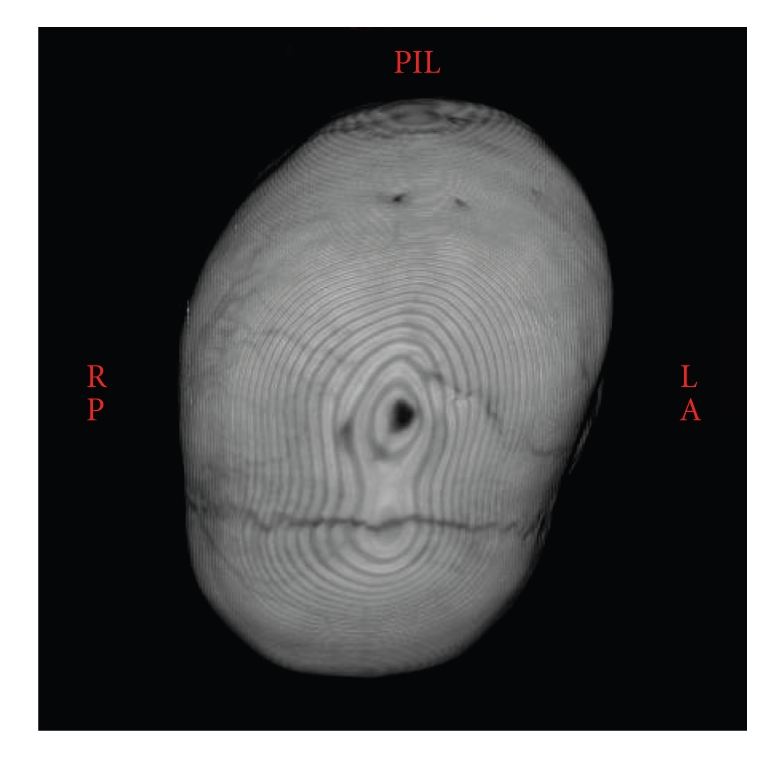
Three-dimensional CT showing the synostotic sagittal suture with a posteriorly twisted skull.
